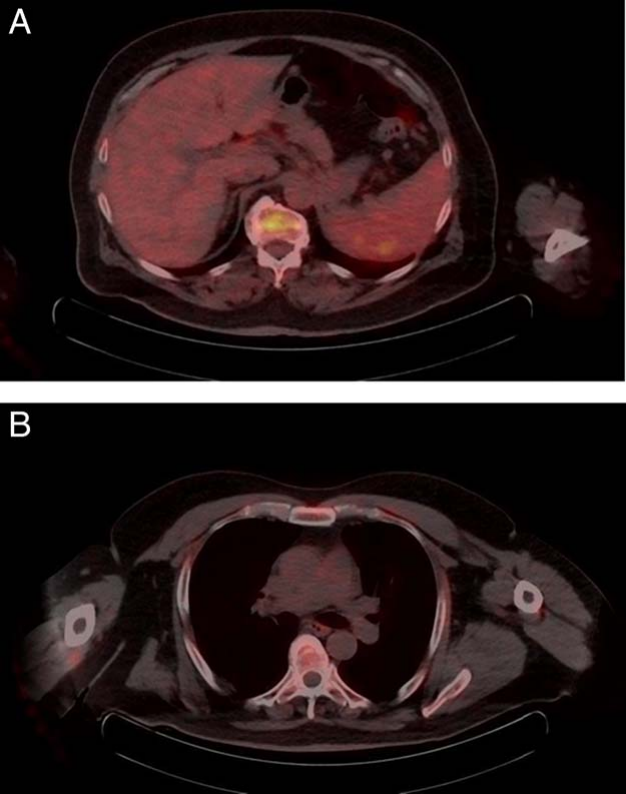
Positron emission tomography after treatment. (A) No definitive hypermetabolic lesion at the anatomical area of the primary lesion. There is slight fluorodeoxyglucose (FDG) activity which is consistent with postoperative changes. (B) Complete resolution of the previously seen hypermetabolic pulmonary nodules.
